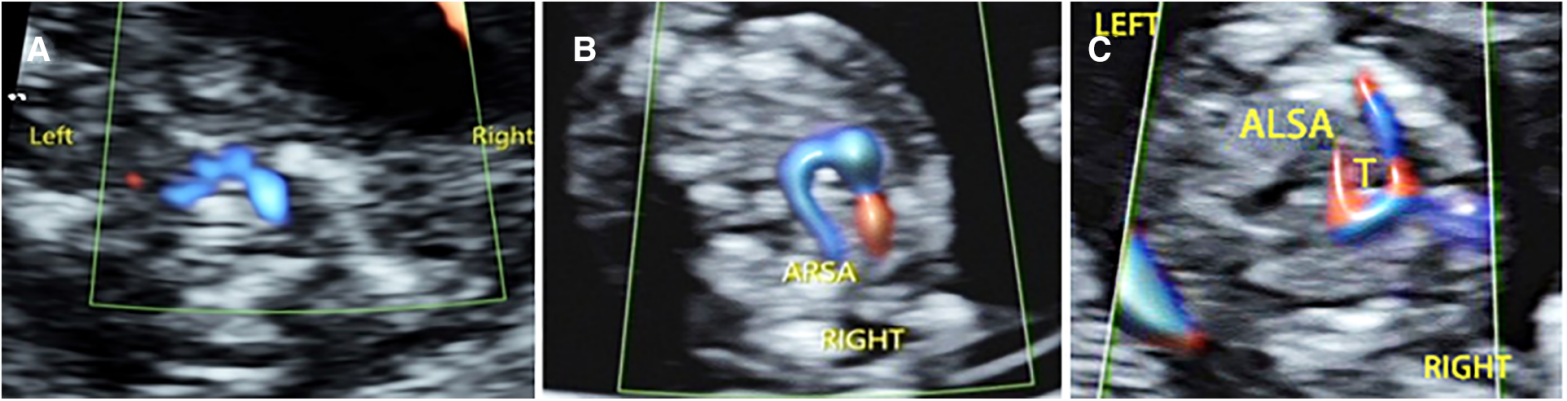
Abnormal ultrasound image pattern of the bilateral subclavian artery section. (A) Normal bilateral subclavian arteries are in front of the trachea, and they form a “bow” structure. (B) BSa-1 pattern: The right subclavian artery originates from the descending aorta, bypasses the trachea, and then runs to the right. (C) BSa-2 pattern shows that the left subclavian artery originates from the descending aorta and is circumvented behind the trachea to the left.
